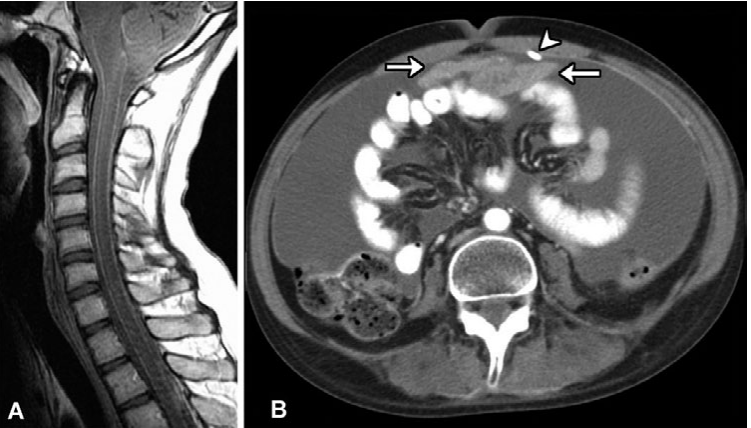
A. Postcontrast sagittal T1-weighted MR image of the cervical spine shows diffuse leptomeningeal enhancement along the surface of the spinal cord. B. Axial contrast-enhanced CT image through the abdomen demonstrates extensive ascites and an enhancing midline mass (arrows) adjacent to the shunt catheter (arrowhead).
